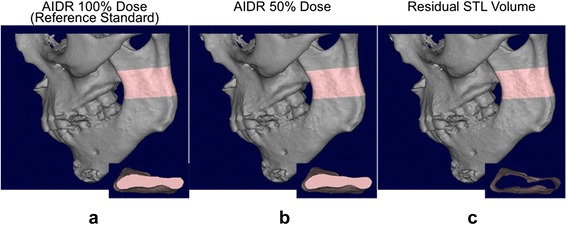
STLs derived from CT images of one patient reconstructed at different mAs (a, b, 100 and 50 % radiation dose, respectively, using the AIDR3D reconstruction algorithm). A portion of the mandible is *highlighted in pink* and the volume enclosed by the STL for that portion is shown in the *inset* from a superior-to-inferior view. Topologic subtraction of the 50 % dose volume is performed to yield the residual volume, i.e., the physical volume occupied by either the 100 or 50 % dose STL but not the other (c)
